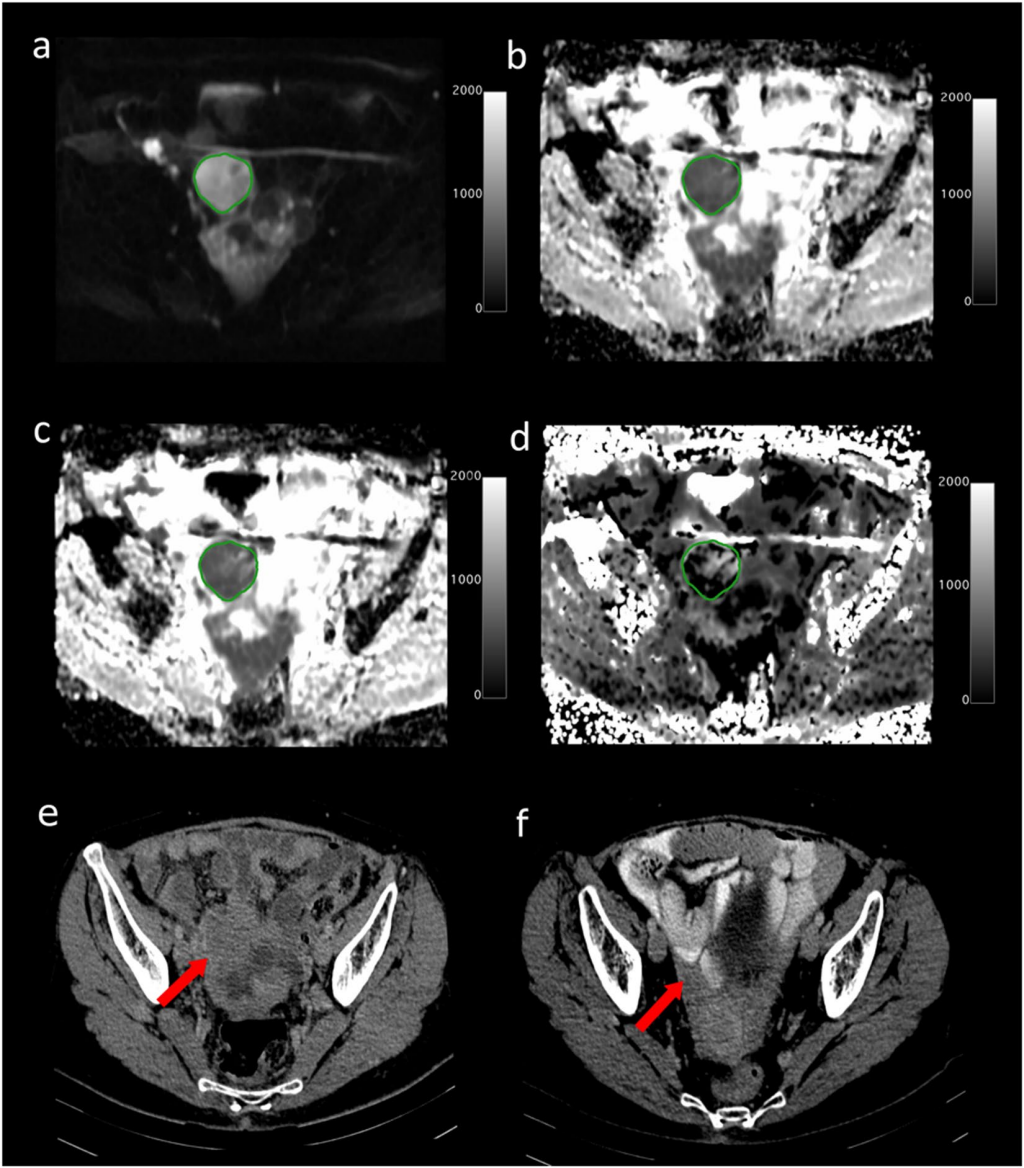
Axial MRI images from a 63-year old high grade serous ovarian cancer patient who had a good response to neo-adjuvant chemotherapy. (a) DWI at b = 1300 s/mm^2^. Scale bar represents signal intensity; (b) ADC map with tumour ROI shown. Scale bar represents ADC in mm^2^/s × 1000; (c) D_app_ map. Scale bar represents D_app_ in mm^2^/s × 1000; (d) K_app_ map. Scale bar represents K_app_ × 1000; Axial CT scans following intravenous contrast medium: (e) before treatment; (f) after treatment, depicting a RECIST Partial Response (PR).
